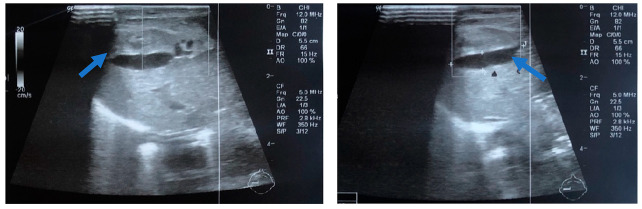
Abdominal ultrasonography depicting a cystic formation 2.1 cm × 0.5 cm in the anterior portion of the right liver, with anechoic characteristics (blue arrow).
